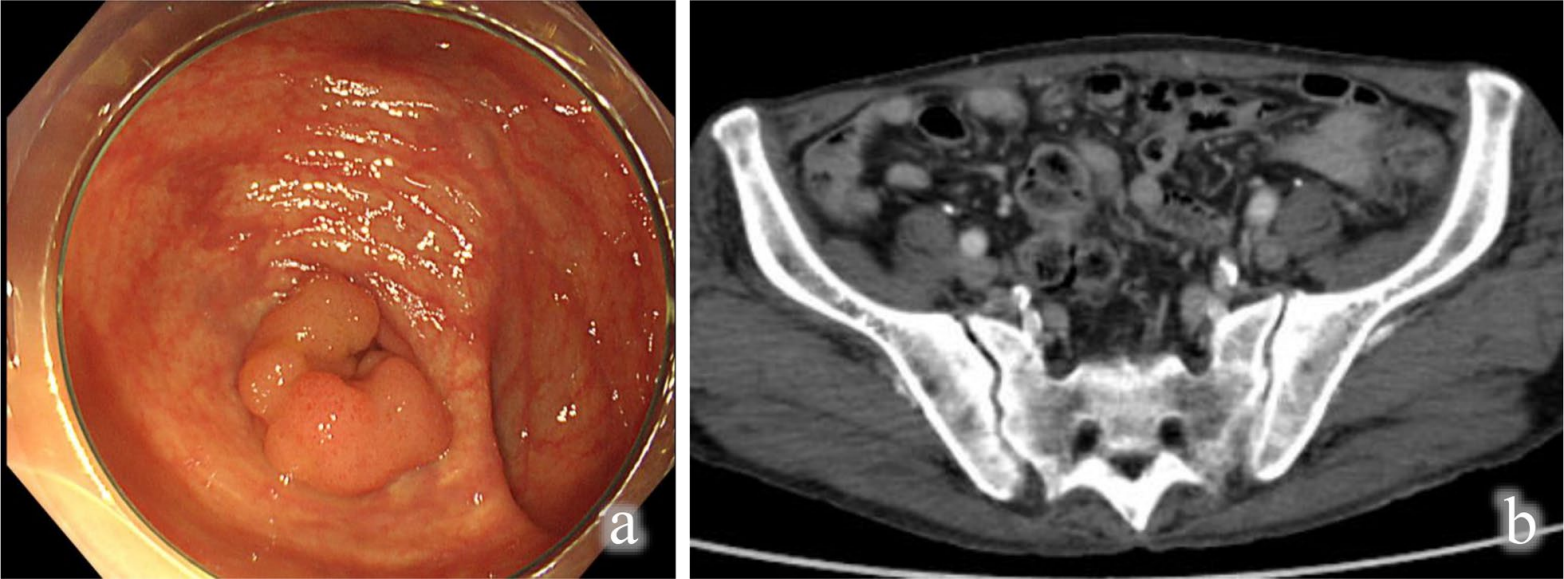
Preoperative examinations. a Colonoscopy findings. A 15-mm sessile serrated polyp was identified near the junction of the cecum and appendix; b computed tomography (CT) showed no metastases to other organs or lymph nodes
